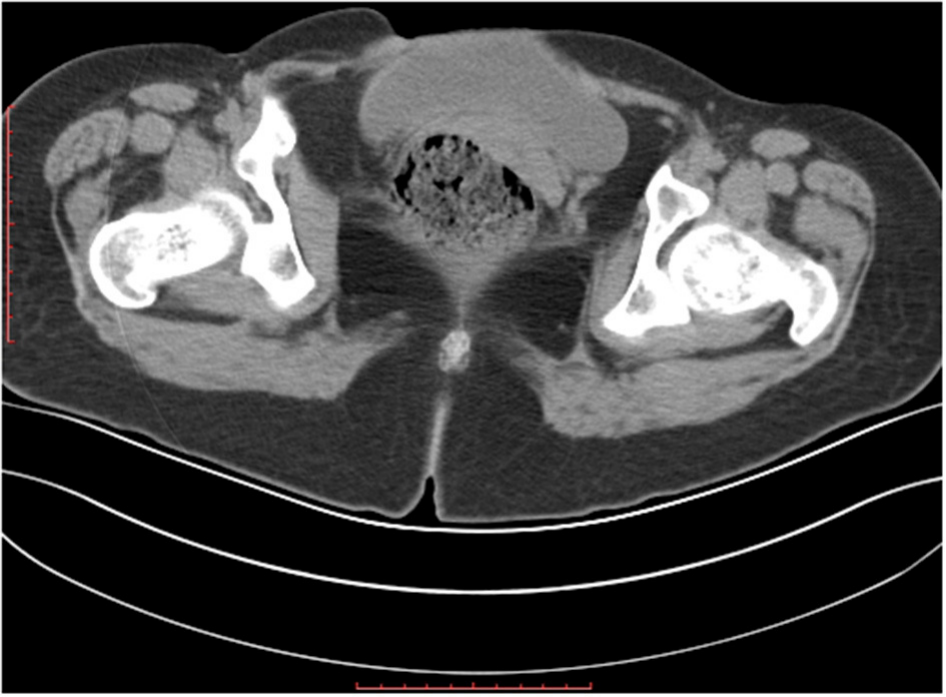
Computed tomography showing the large fascial defect on the axial slices.
